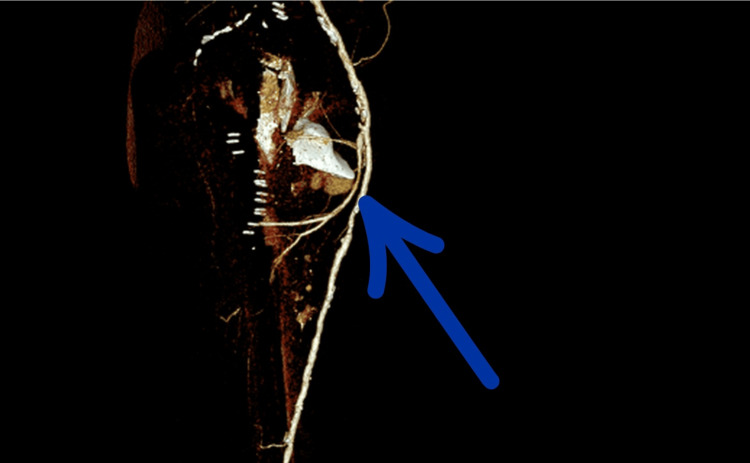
CT angiography of the left lower limb showing a large pseudoaneurysm of the profunda femoris artery, in close anatomical proximity to the displaced lesser trochanter fragment (right side view). Direct contact between the displaced lesser trochanter fragment and the profunda femoris artery is clearly visible.
